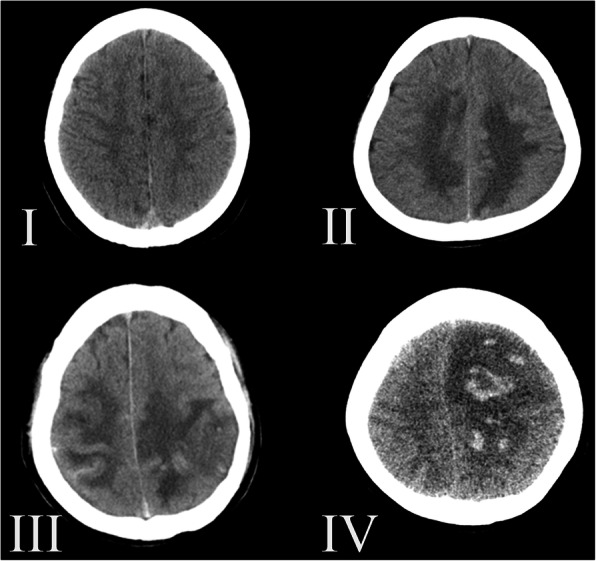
CT image-based classification and grading system: I no obvious abnormality (grade I); II simple vein infarction without hemorrhage (grade II); III cerebral venous infarction with subarachnoid hemorrhage (grade III); IV cerebral vein infarction with hematoma (grade IV)
